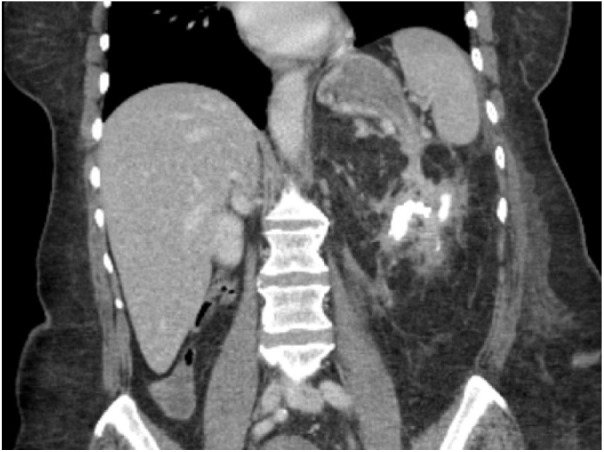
Computed tomography scan of abdomen and pelvis with intravenous contrast
